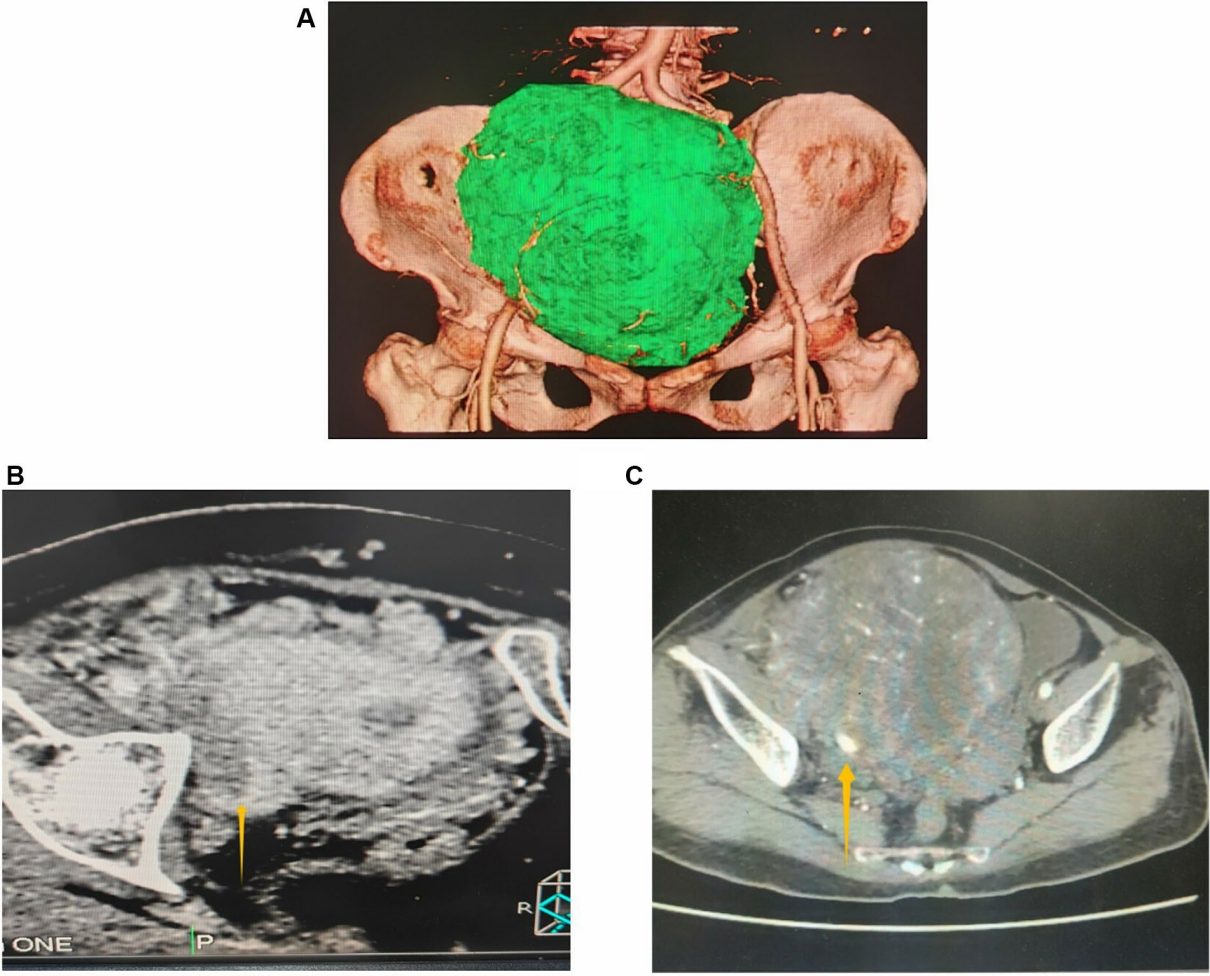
(A) Three-dimensional imaging based on CT scan shows a huge abdominal mass; (B,C) contrast agent-enhanced CT shows the cross-sectional view of the mass. The arrow (B) points to the external iliac vein; the arrow (C) points to the external iliac artery.
